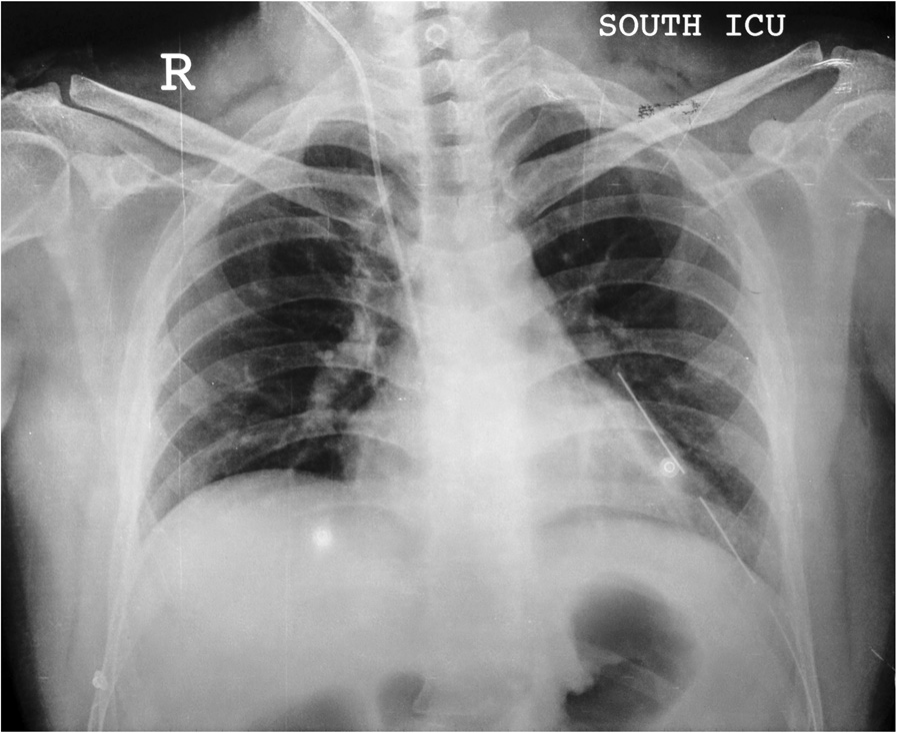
X-ray chest showing full expansion of the left lung with IJV catheter and ICD tube in situ
